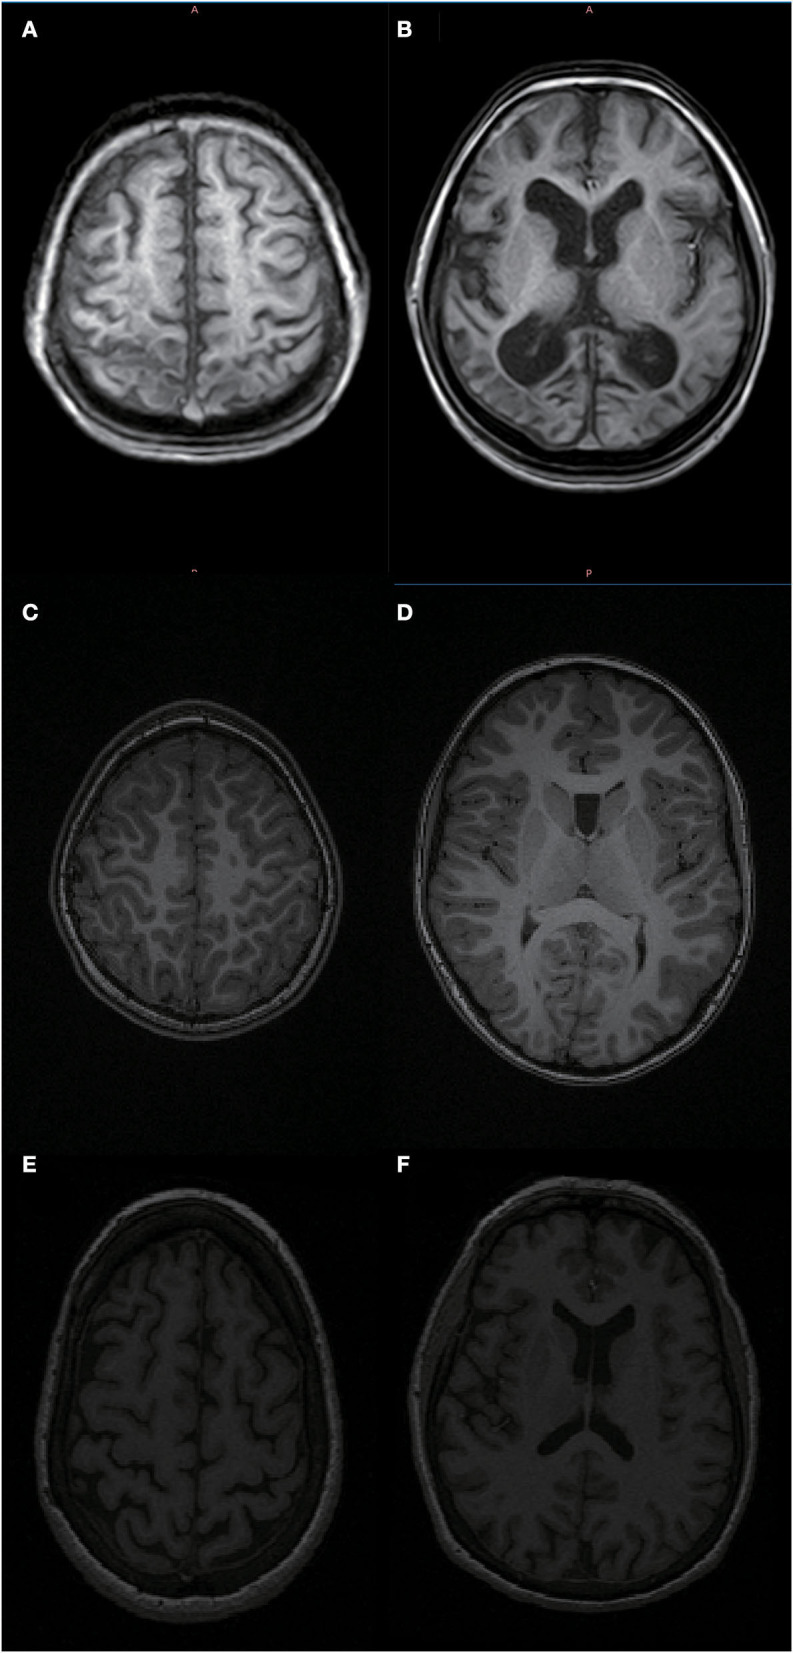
MRI of an 11-year-old patient with bilateral spastic cerebral palsy vs. an 11- and 62-year-old healthy person. As shown in (A,B) the head MRI of an 11-year-old patient with bilateral spastic cerebral palsy, who has significant brain atrophy and the patient's brain age is 61.74 years; (C,D) the head MRI of an 11-year-old healthy person; (E,F) the head MRI of a 62-year-old healthy elderly person. Based on the pictures, we can see that the 11-year-old bilateral spastic cerebral palsy patient's degree of brain atrophy is relatively close to that of a 62-year-old healthy elderly person.
